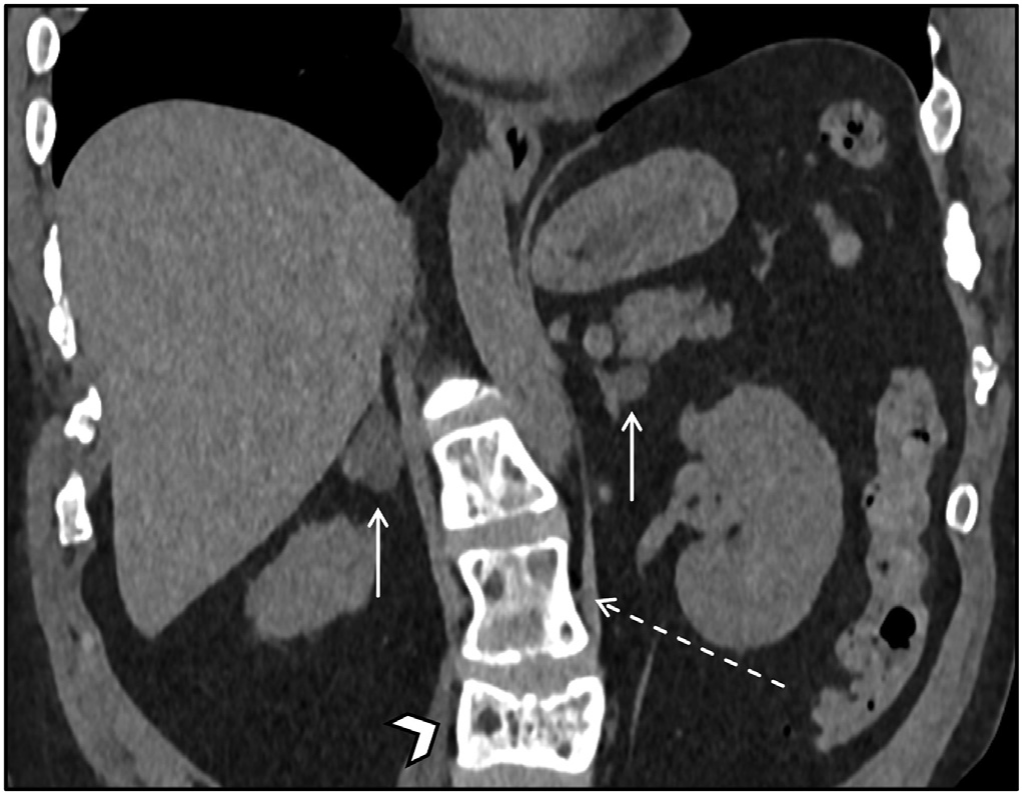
Bilateral macronodular adrenal hyperplasia in McCune-Albright Syndrome. Coronal noncontrast CT image demonstrates diffuse nodular enlargement of the adrenal glands bilaterally with homogeneous low-density lesions (arrows) consistent with bilateral nodular adenomatous hyperplasia in the setting of McCune-Albright Syndrome. Note diffuse bone abnormalities in keeping with multiple areas of fibrous dysplasia, with bone expansion and ground glass appearance (dashed arrow), variable rib spacing, and a vertebral compression deformity (arrowhead). *CT* = computed tomography.
